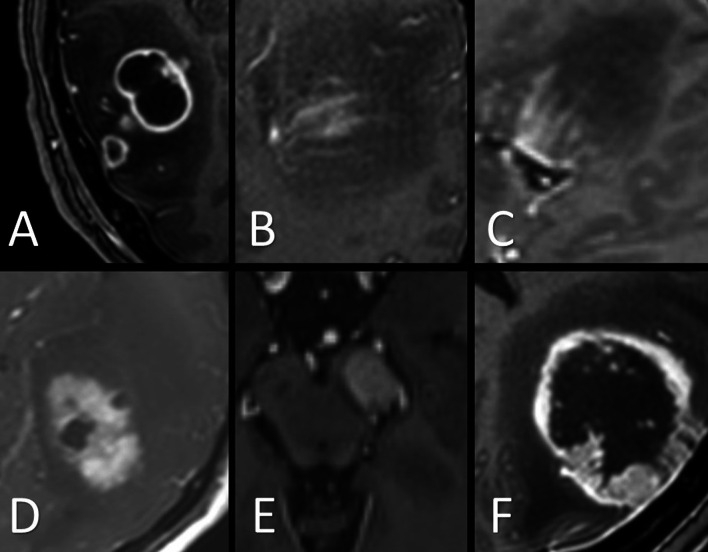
Example of lesion patterns in contrast-enhanced T1-weighted sequences: A cystic B stripe-like C tree-like D mixed solid / cystic E) solid F) solid with central necrosis, counted as mixed solid / cystic. Gb lesions (A, D, F), CNS lymphoma lesions (B, C, E)
